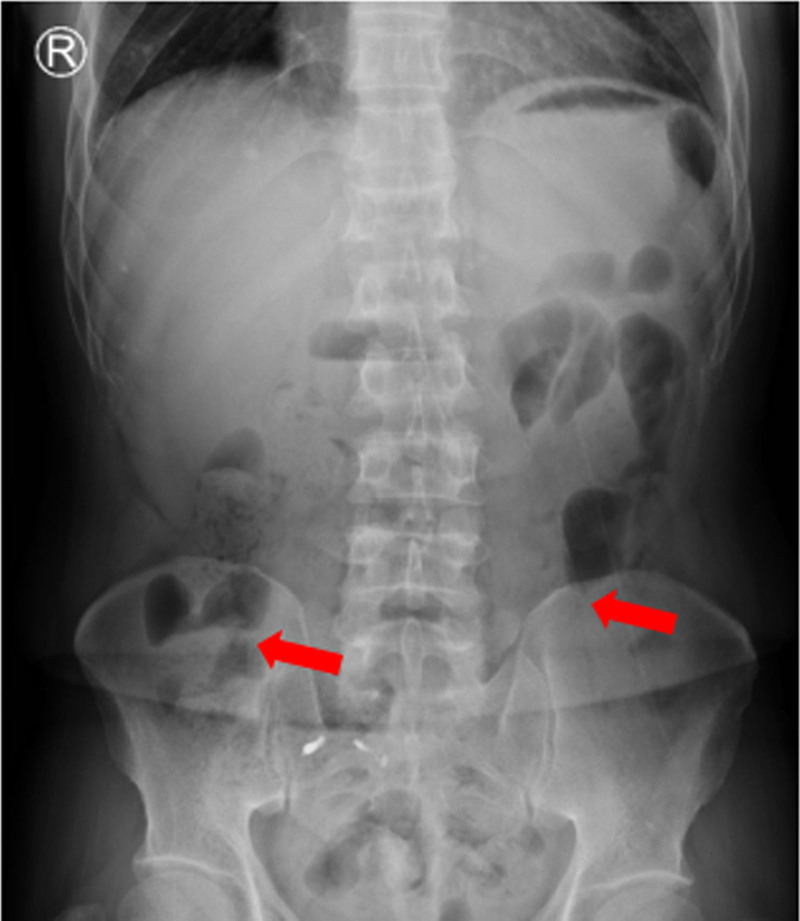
Chest abdominal X-ray showed intestinal gas and fluid accumulation (red arrows).
